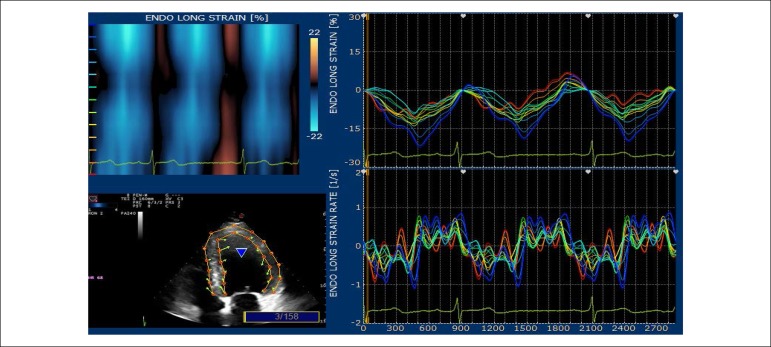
Apical four-chamber – percentage of longitudinal strain in the basal, middle and apical segments of the inferior and anterolateral septal walls.
